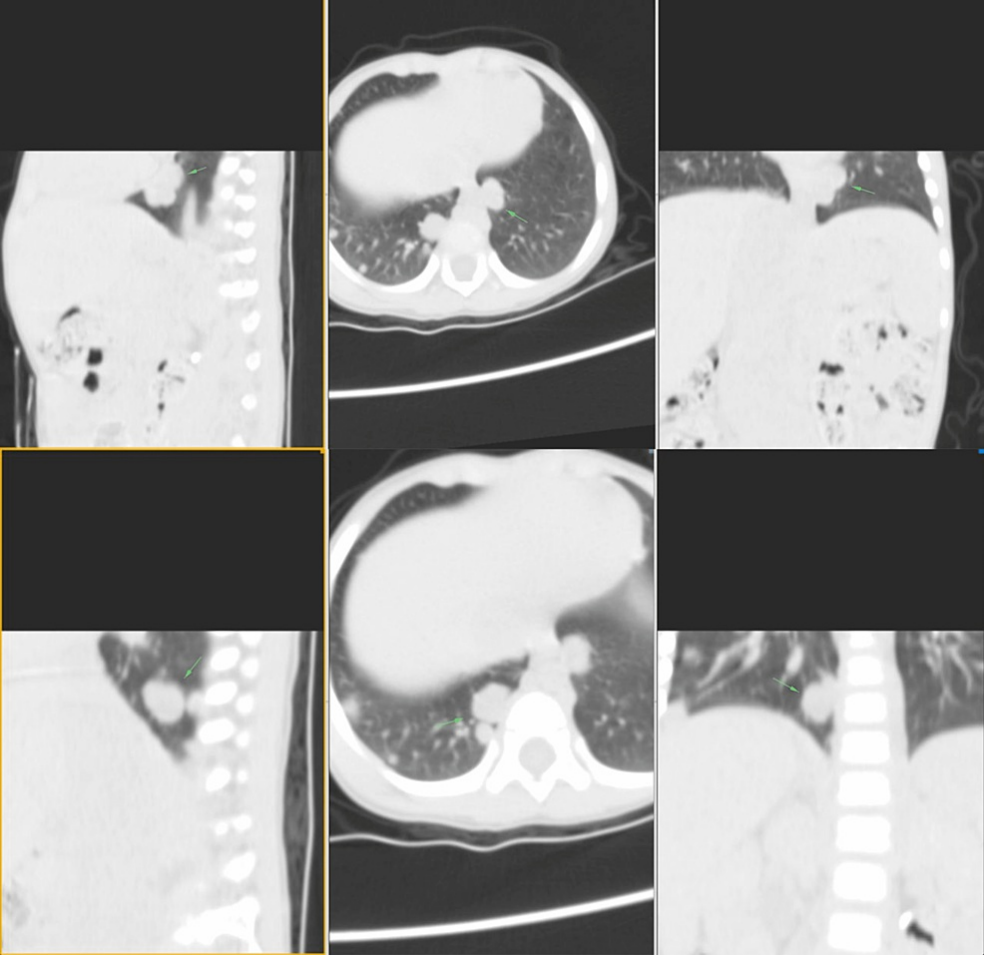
MSCT showed bilateral nodular-type pulmonary metastases. The green arrow showed hyperdense lesion in bilateral pulmonary inferior lobe visualized as nodule with firm borders, regular edges, diameter of 1.1 cm, with increased post-contrast density. MSCT, multislice computed tomography
